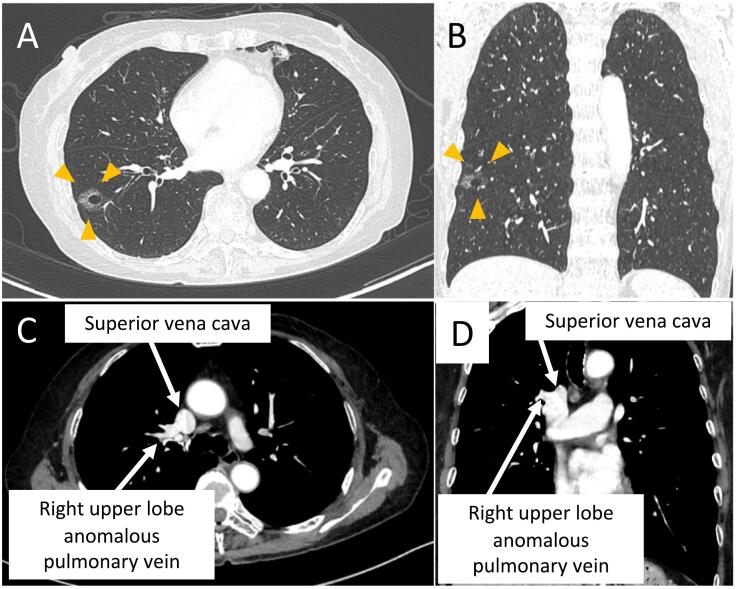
Computed tomography images. (A, B) Thoracic computed tomography showed a tumor shadow indicated by yellow arrows in the right lower lobe and (C, D) an enhanced anomalous pulmonary vein. (For interpretation of the references to colour in this figure legend, the reader is referred to the web version of this article.)
